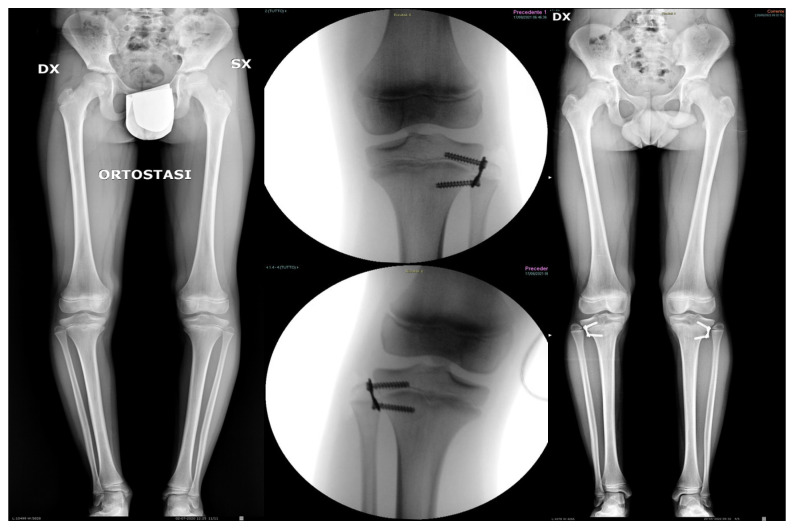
Pre-operative, intraoperative, and post-operative radiographs of an 11-year-old male patient with idiopathic genu varum. The plates were removed after 9 months, showing good restoration of the mechanical axis with slight intentional overcorrection (increased MPTA).
